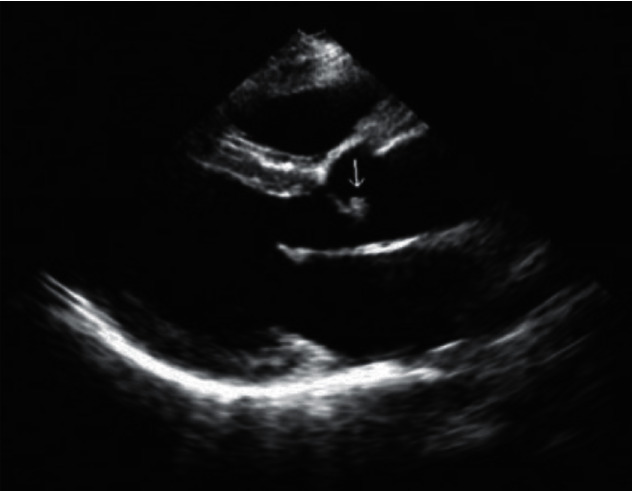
Transthoracic echocardiogram demonstrated the vegetation locating in the aortic valve (white arrow).
